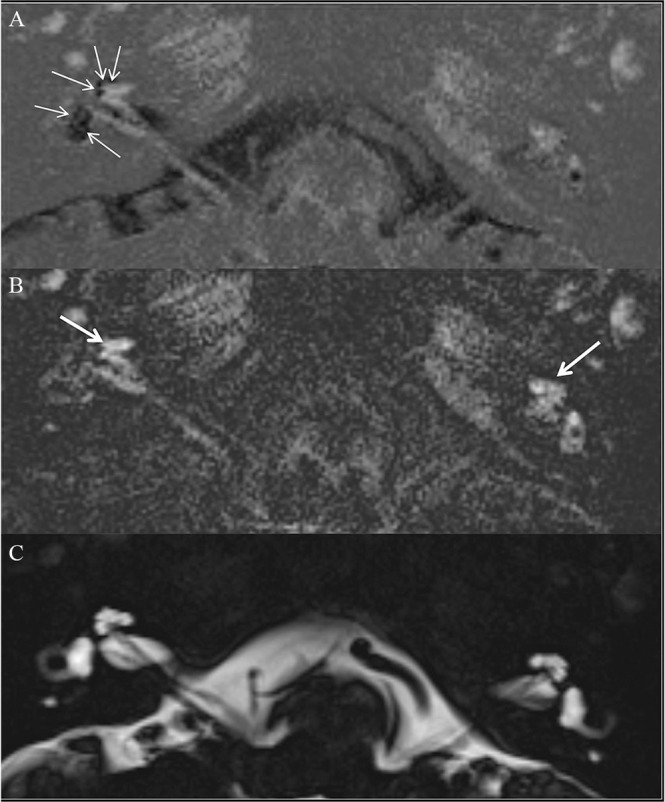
A 28-year-old woman with right Ménière’s disease. (A) HYDROPS2 image shows significant endolymphatic hydrops in the cochlea and vestibule (arrows). (B) Heavily T_2_-weighted three-dimensional fluid-attenuated inversion recovery (3D-FLAIR) image obtained 4 hours after intravenous administration of a single dose of gadolinium-based contrast agent (IV-SD-GBCA). High signal is seen bilaterally in the cochleae (arrows). In this particular subject the signal intensity ratio (SIR) was 11.7 in the right cochlea and 7.5 in the left cochlea. (C) Magnetic resonance cisternography (MRC) at the slice level corresponding to that in (B).
